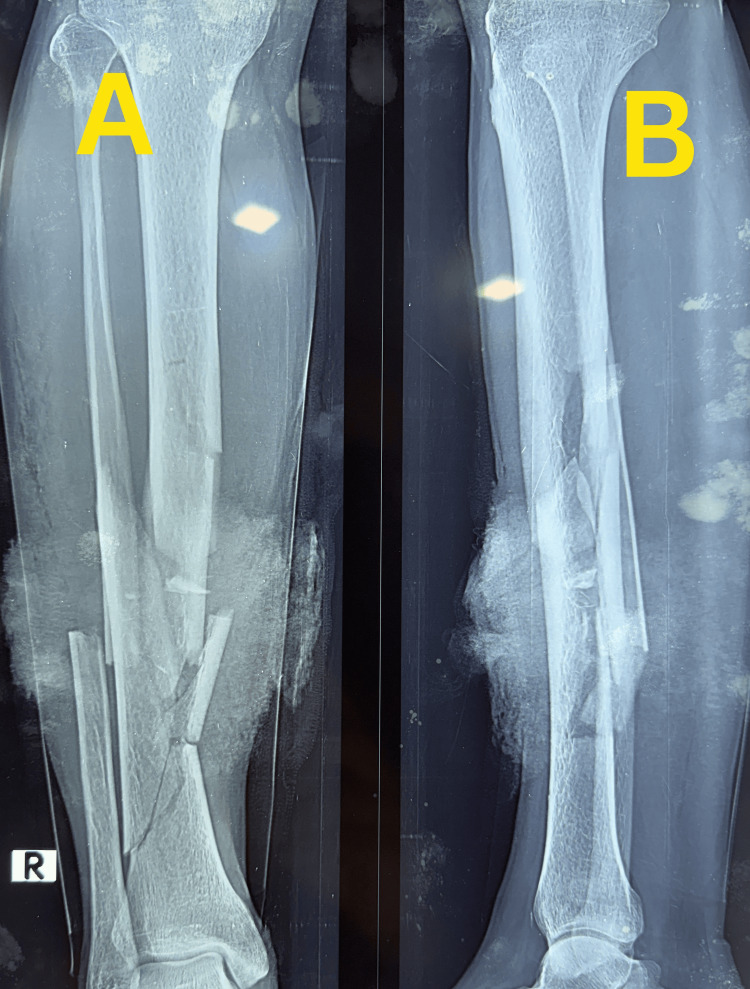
Preoperative radiographs of the right leg Preoperative anteroposterior (A) and lateral (B) radiographs of the right leg obtained on 14 November 2025, demonstrating a segmental comminuted fracture of the tibia with an associated ipsilateral mid-shaft fibula fracture.
